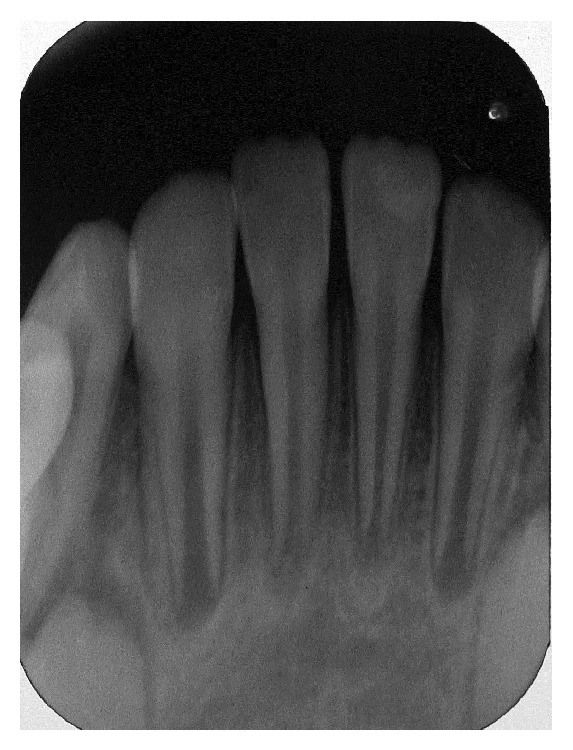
Six-month radiographic follow-up. Closure process of the apexes of the traumatized teeth continued normally.
